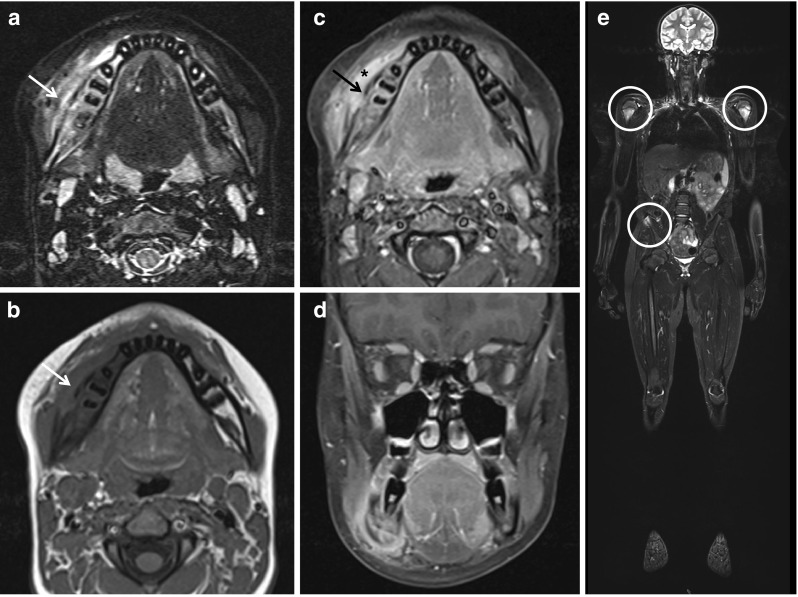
Magnetic resonance imaging in CNO/CRMO. Magnetic resonance imaging in a 15-year-old male patient with swelling and warmth over right mandible. a Transversal TIRM sequences unveiled bone swelling and edema of the right mandible (arrow). b Native and c contrast-enhanced transversal T1 sequences with fat saturation unveiled new bone formation (arrows), resulting swelling, and in c enhancement in the right mandible (asterisk). d Coronary T1 sequences with fat saturation in the same patient. e Whole body MRI (coronary TIRM sequences) unveiled additional sites of bone inflammation at both proximal humeri and the right upper iliac spine (circles) (MRI images with friendly permission from Gabriele Hahn, Pediatric Radiology, Medizinische Fakultät Carl Gustav Carus, Technische Universität Dresden, Dresden, Germany)
